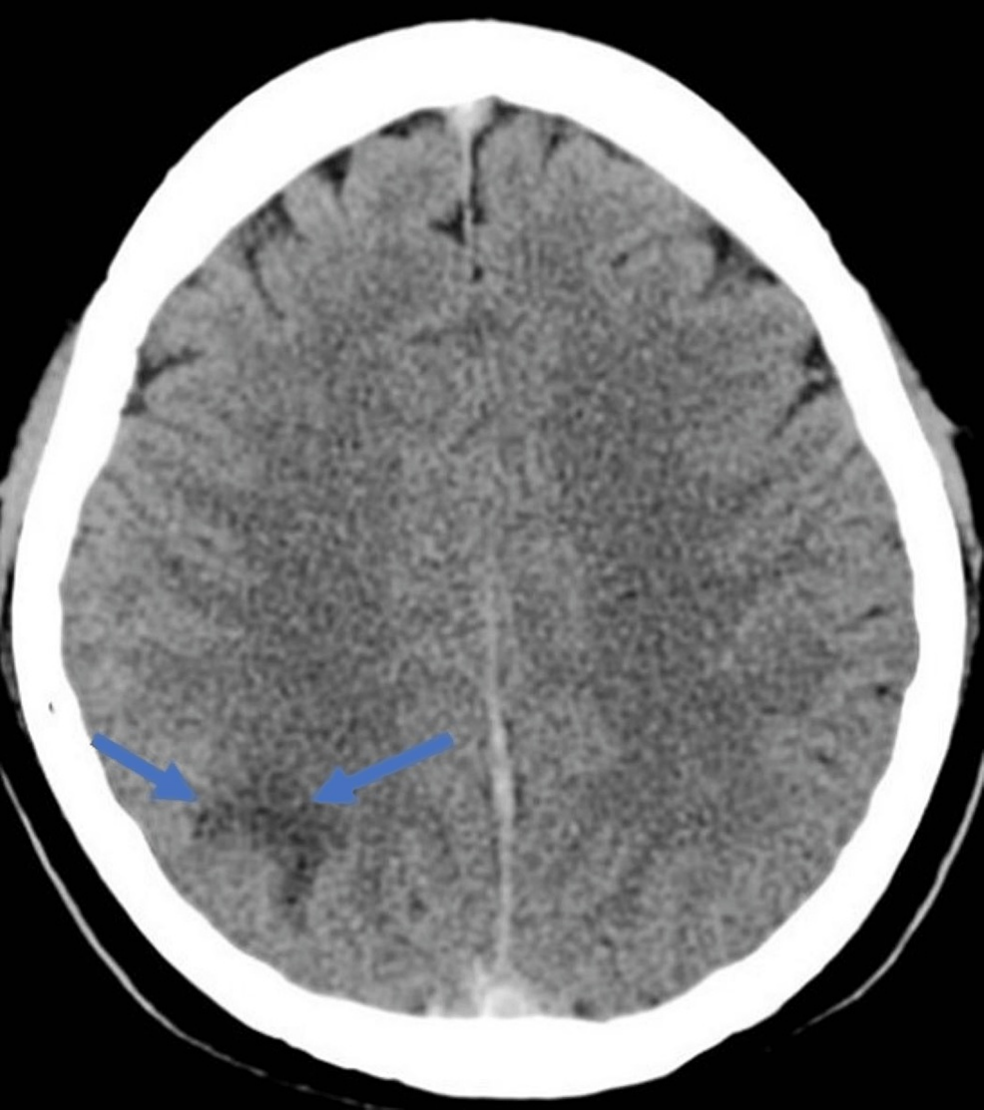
Computed tomography showing a region of low density of hemorrhagic focus in the right parietal lobe.
